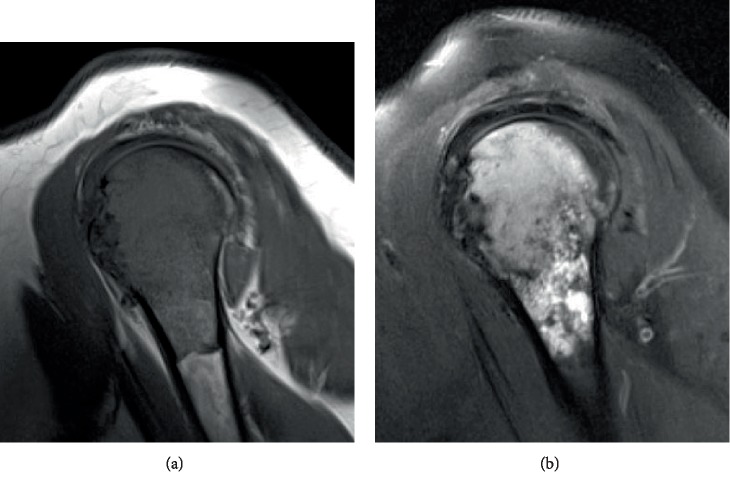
Case 1: sagittal left shoulder MRI showing a 6 cm intramedullary lesion involving the proximal left humerus with associated periostitis. The lesion was hypointense on T1-weighted images (a) and hyperintense on T2-weighted images (b).
